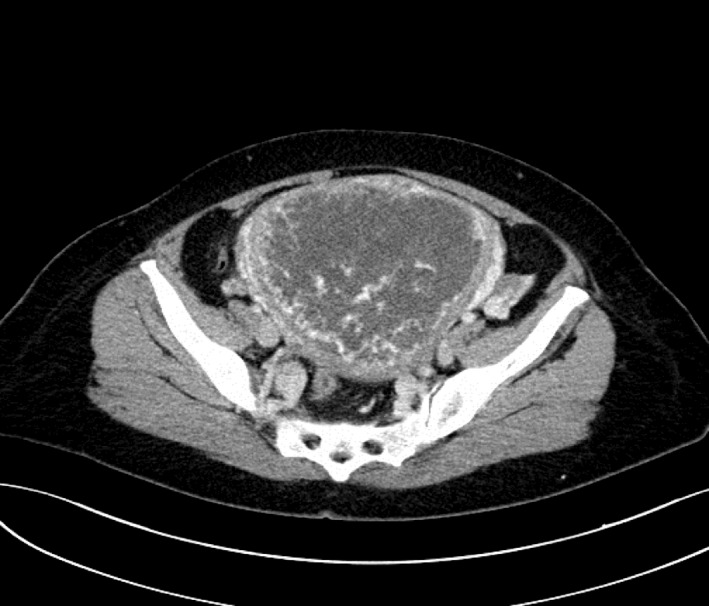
Thoracoabdominopelvic CT scan: Pelvic mass of 14 cm
